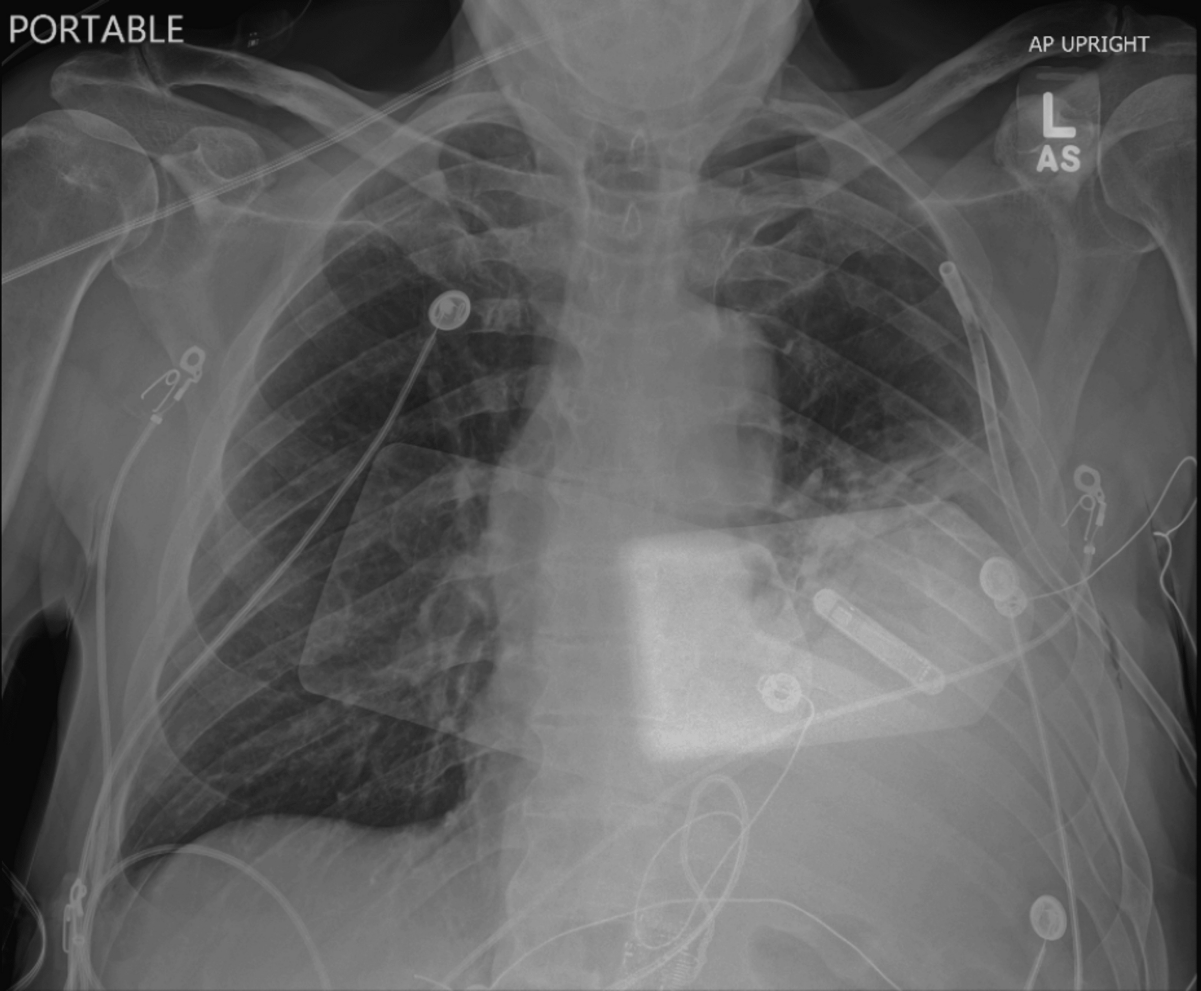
Chest radiography after pigtail chest tube insertion Chest radiography showing improvement in hydropneumothorax and placement of a pigtail chest tube in the left hemithorax with normal tracheal positioning.
